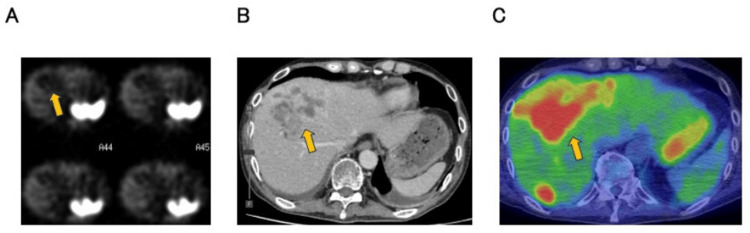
SSTR scintigraphy, contrast-enhanced CT, and FDG-PET. (A) SSTR scintigraphy demonstrated physiological uptake in the spleen and the upper pole of the right kidney, but no abnormal uptake corresponding to the tumor lesions identified on contrast-enhanced CT and FGD-PET.​ (B) Contrast-enhanced CT revealed multiple hepatic masses in S5, characterized by peripheral ring-like enhancement. (C) On PET-CT, intense FDG uptake was observed in the hepatic lesions corresponding to those identified on contrast-enhanced CT. Physiological uptake was also noted in the upper pole of the right kidney. PET: positron emission tomography; CT: computed tomography; FDG: fluorodeoxyglucose; SSTR: somatostatin receptor scintigraphy.
